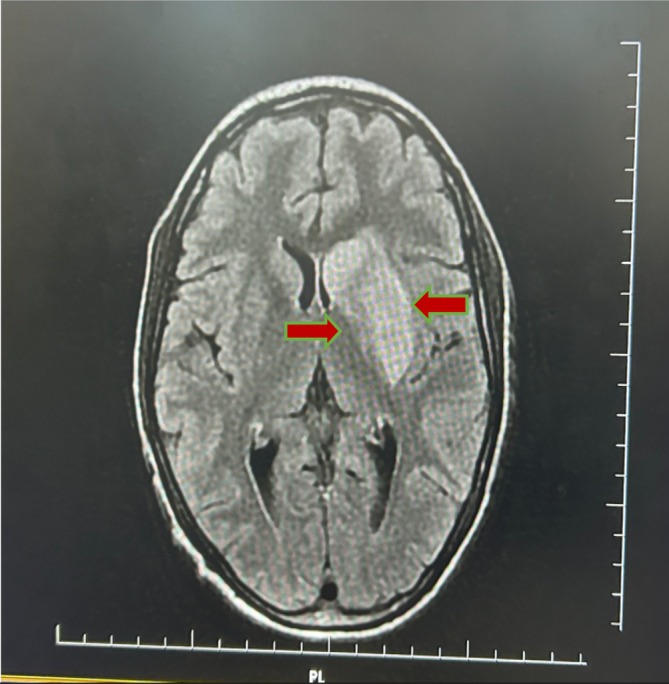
Brain MRI Scan T2 FLAIR revealed a hyperintense (bright) region in the left basal ganglia.
